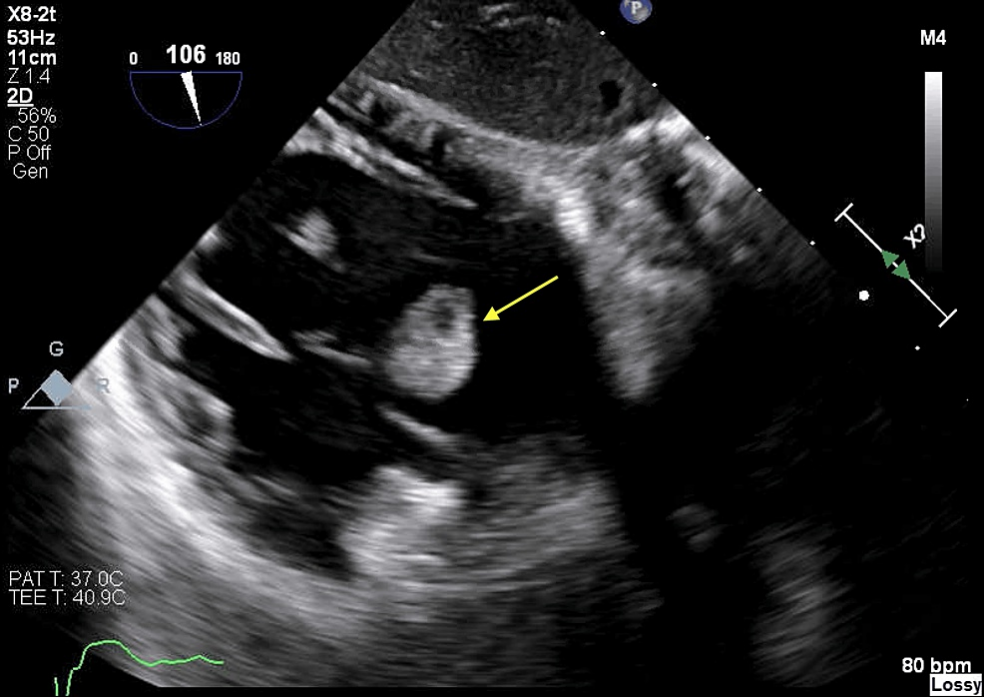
Transesophageal echocardiogram A large (1.6 x 1.7 cm) round echo dense mass observed on the atrial side of the tricuspid valve is highlighted by a yellow arrow in the TEE image. TEE: Transthoracic echocardiogram
